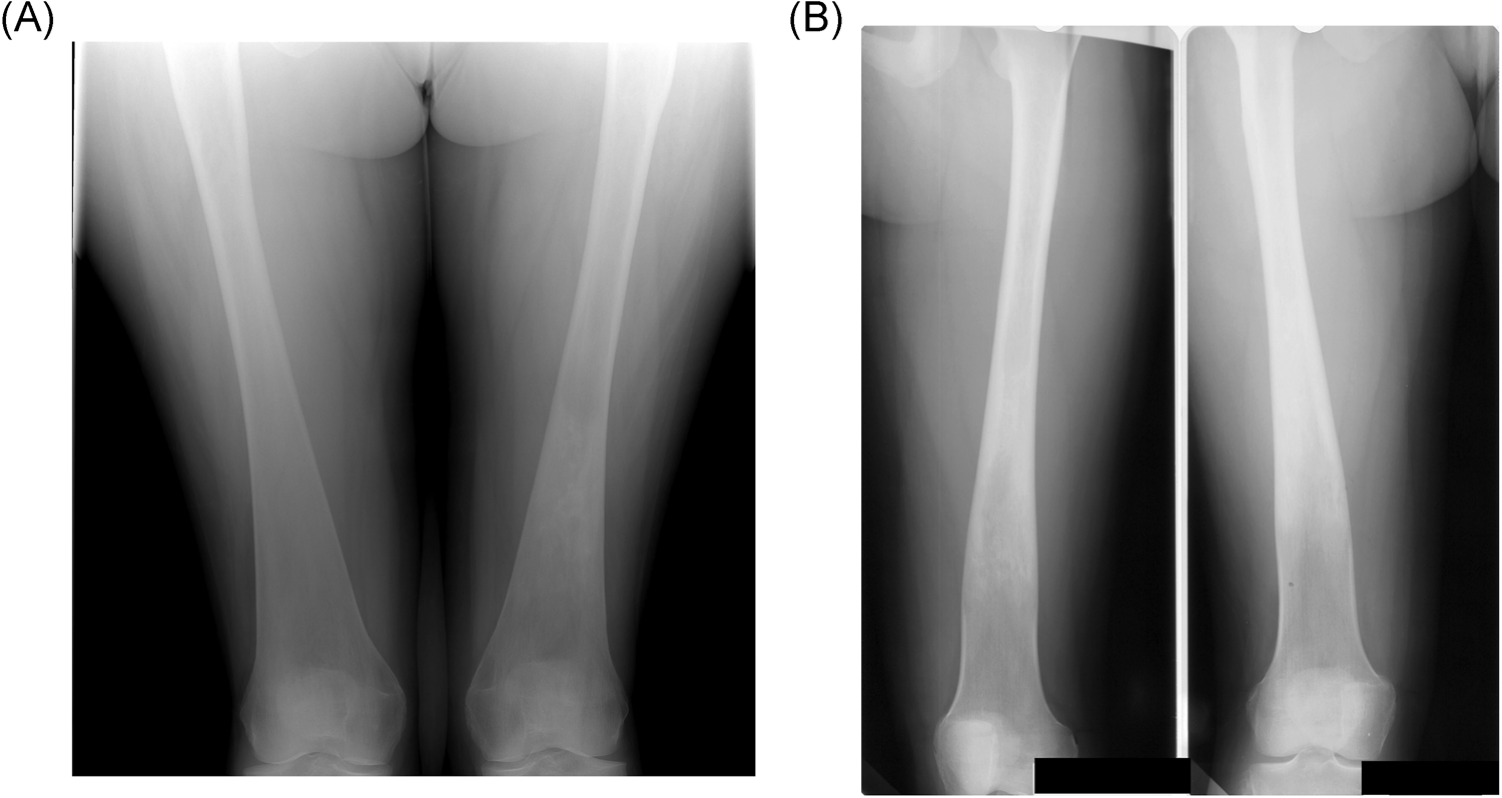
Erlenmeyer flask deformity. (*A*) Typical appearance. Radiograph of the lower femora, showing the triangular outline of the metaphysis. Note the indistinct boundary between the cortex and medulla, typical of the Erlenmeyer flask deformity in Gaucher disease (GD). Incidentally, there is an area of serpiginous sclerosis in the left femoral metaphysis, suggestive of osteonecrosis. (*B*) Atypical appearance. Radiograph of the lower femora of a woman with GD who began enzyme replacement therapy at the age of 12 years. The proximal metaphysis has features similar to those in *A*, but the distal metaphysis has the more normal, trumpet‐shaped outline of the distal femur, with a clear border between the cortex and medulla. We speculate that the normal modeling process of endochondral ossification took place from the time of initiation of therapy
